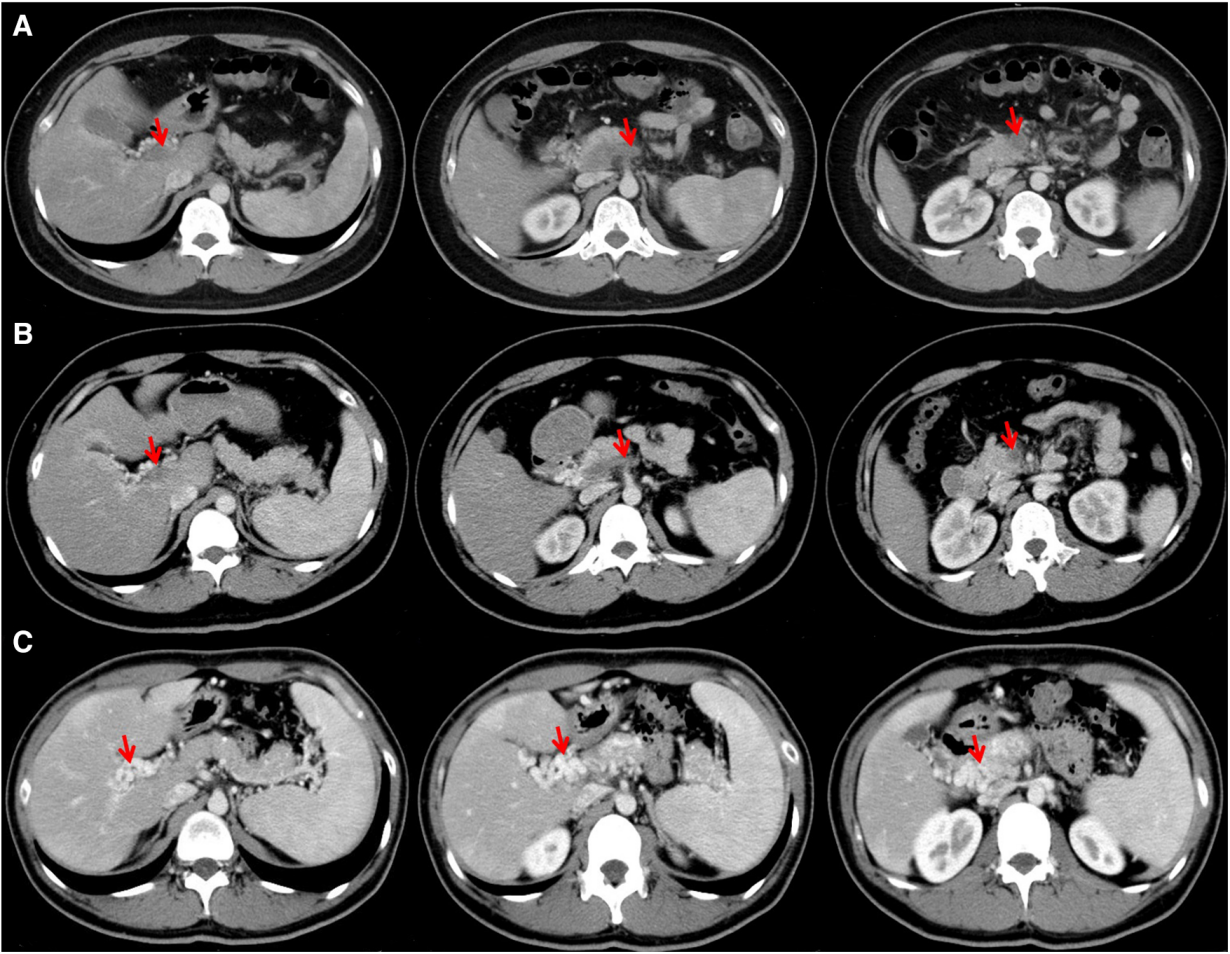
Contrast-enhanced CT images performed on July 17 (A) and July 26 (B) showing thrombosis in portal vein, splenic vein, and superior mesenteric vein, and those on January 5, 2024 (C) showing cavernous transformation of the portal vein.
